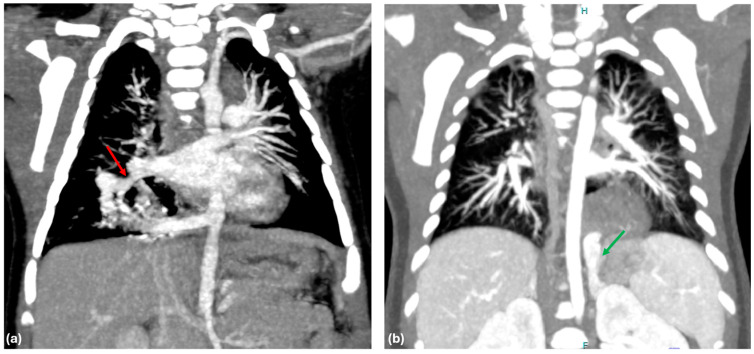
(a) CT scan showing a right lower ILS draining in to right inferior pulmonary vein (red arrow: pulmonary venous drainage); (b) CT scan showing a left lower ELS draining into the left adrenal vein (green arrow: systemic venous drainage).
